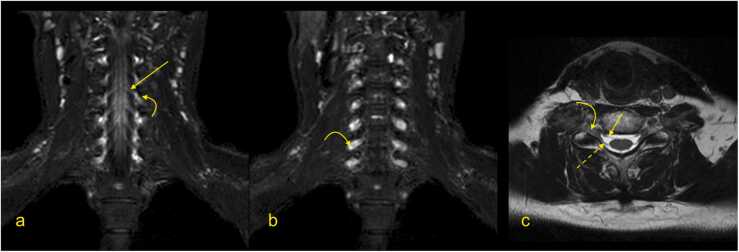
Magnetic resonance imaging of the brachial plexus. (a and b) T2-weighted DIXON sequence with fat suppression, (c) T2-weighted turbo spin-echo sequence). Curved arrow – the ganglion of the spinal nerve, arrow – the anterior root of the spinal nerve, dashed arrow – the posterior root of the spinal nerve.
